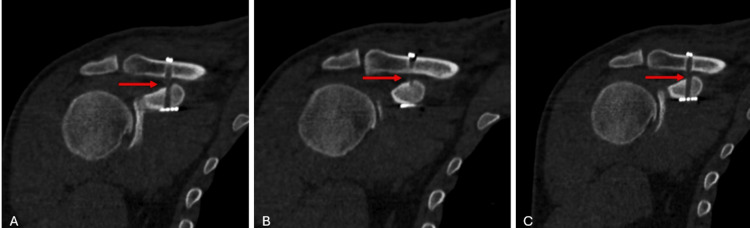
Postoperative coronal CT images of the patient The images show the tunnel placement in the coracoid bone and clavicle, confirming proper implant positioning within the tunnel. The red arrows indicate the tunnel.
